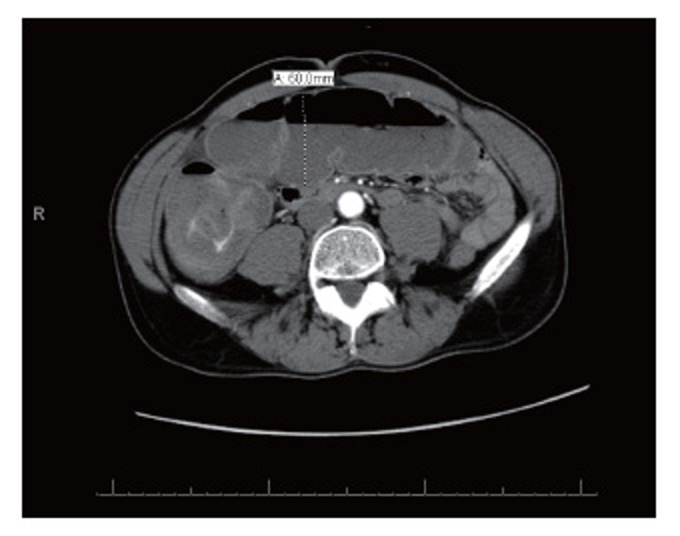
CT with oral and IV contrast on presentation. Transverse colon dilated to 6 cm with collapse of large bowel distal to splenic flexure. There is no obvious identifiable mass.
